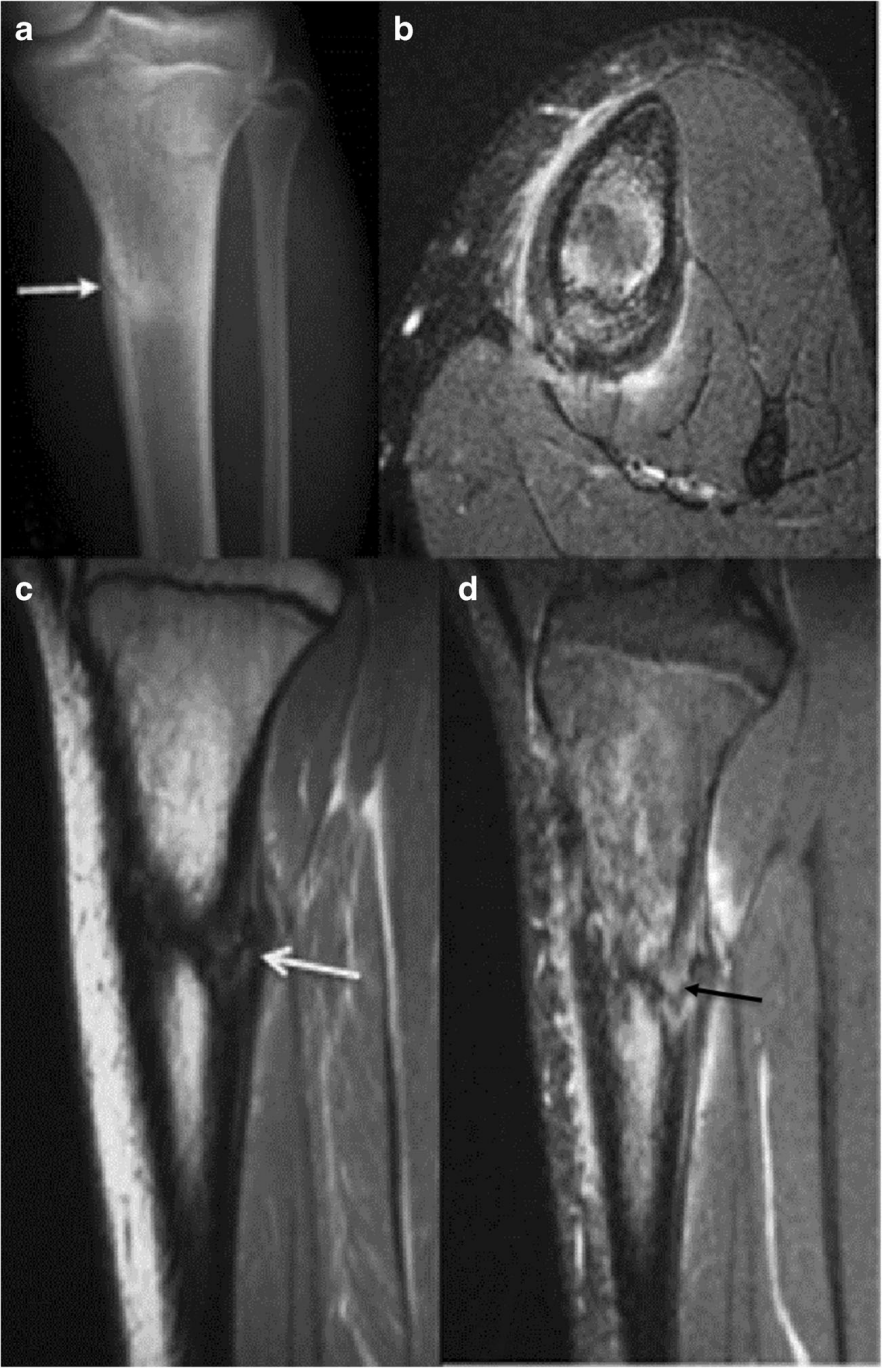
Periosteal reaction post trauma. An antero-posterior radiograph of the leg bones a shows a healing fracture at the postero-medial aspect of the tibia. Adjacent cortical thickening and surrounding sclerosis are seen (*arrow*). Axial T2 b coronal T1 c and coronal T2 d images demonstrate a hypointense healing fracture line with the surrounding bone and soft-tissue edemaMultilamellated/“onion skin” periosteal reaction is seen most commonly with aggressive tumours such as Ewing’s sarcoma and osteosarcoma, and can also be seen with histiocytosis.Solid periosteal new bone is the hallmark of benign, slow, persistent lesions such as osteoid osteomas. Periosteal reaction associated with osteomyelitis and Langerhans’s cell histiocytosis may be either be single-layered, multilamellated or solid [21].Spiculated periosteal reaction can present as “hair-on-end”, divergent or sunburst patterns and generally occurs with more rapidly unfolding processes such as aggressive malignancies including osteosarcoma, Ewing’s sarcoma or neuroblastoma.Codman’s triangle is an interrupted type of periosteal reaction, which is accompanied by a cuff of reactive bone at the extreme ends of a lesion [21].-Diffuse periosteal reaction:A discussion about periosteal pathology is incomplete without a brief overview of some systemic conditions, which cause widespread periosteal reaction.Infantile cortical hyperostosis (Caffey’s disease) is a self-limiting inflammatory disorder of unknown aetiology affecting infants less than 5 months [28]. Common findings include soft-tissue swelling (frequently over the mandible), fever, hyperirritability, and cortical hyperostosis (ribs, ulna, tibia, fibula, humerus, femur, radius, metacarpal and metatarsal bones). Imaging findings on radiographs and MR include smooth, wavy, and/or irregular diaphyseal or metaphyseal periosteal and cortical thickening affecting one or multiple bones.Diffuse, symmetric periostitis may also occur as a complication of long-term prostaglandin therapy which is administered to maintain ductus arteriosus patency in infants with congenital heart disease (Fig. 18) [29]. Such periostitis is associated with extremity swelling and improves on cessation of prostaglandin infusion [30].
